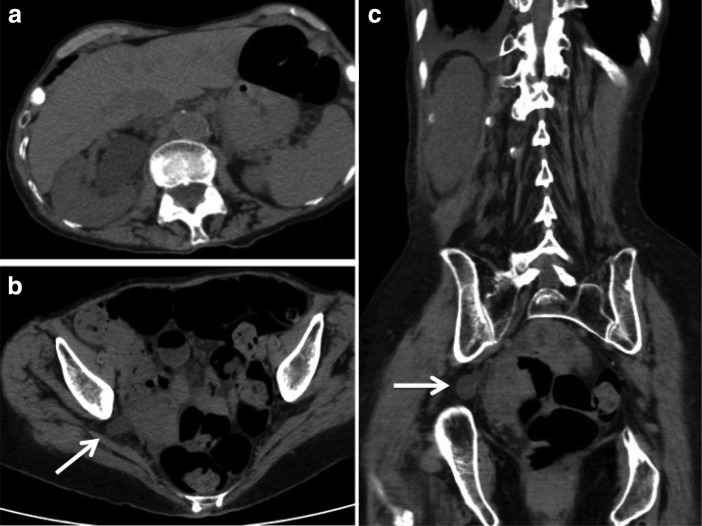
CT scan demonstrating hydroureteronephrosis secondary to herniation of the right ureter into the greater sciatic foramen. (a) Shows hydronephrotic renal pelvis and collecting system and (b, c) demonstrate the dilated ureter within the greater sciatic foramen (marked by *arrow*).
